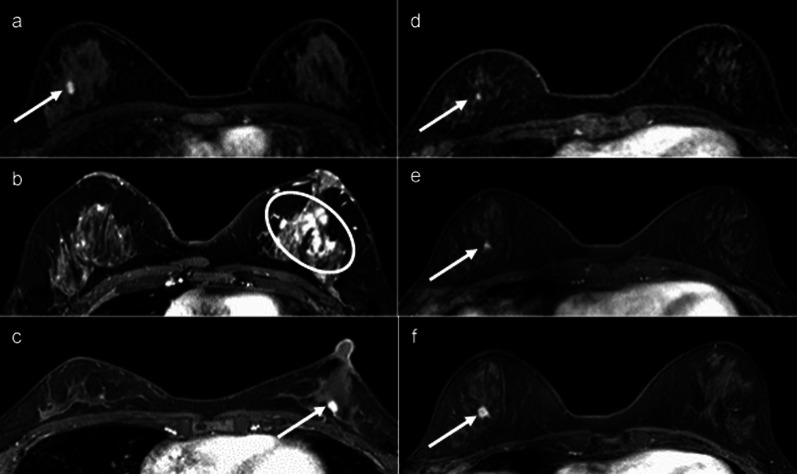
Fat-suppressed post contrast T1-weighted image. a A 9-mm oval circumscribed mass (arrow of a) on Case 1. b Segmental clumped non-mass enhancement (circle of b) on Case 2. c A 6-mm round circumscribed mass on the chest wall side (arrow of c) on Case 3. d A 3-mm enhanced nodule (focus) (arrow of d) on Case 4. e Three months later from first screening MRI, no significant change (arrow of e) on Case 4. f Nine months later from first screening MRI, the lesion was increased as a 10-mm not-circumscribed and heterogeneous enhanced mass (arrow of f) on Case 4
